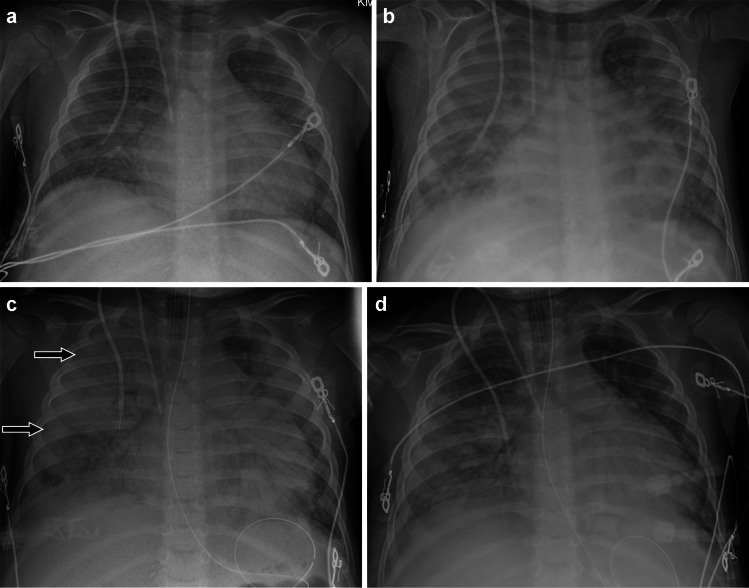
A 3-year-old male with neuroblastoma who developed acute bronchospasm during dinutuximab (a monoclonal antibody targeting glycolipid GD2) infusion and subsequent respiratory failure requiring intubation in the setting of clinical evidence of capillary leak. Infection work-up was negative. a-d Anteroposterior chest radiographs obtained over the course of 4 days showing rapid development of widespread bilateral consolidation and a right pleural effusion (*black arrows*), and subsequent rapid decrease in findings. The temporal relationship of the respiratory condition to dinutuximab infusion and the imaging findings support the diagnosis of dinutuximab-induced capillary leak syndrome, an adverse effect known to occur with dinutuximab
